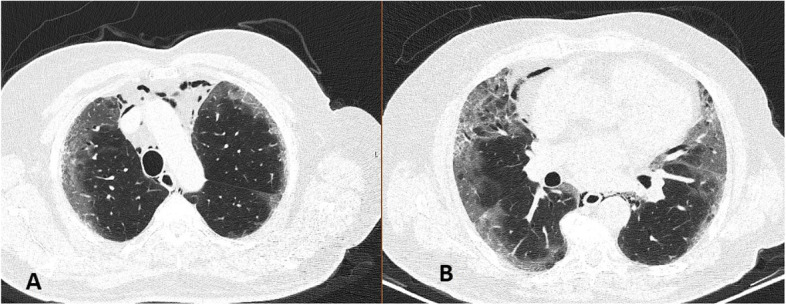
Chest CT obtained at presentation to the ED in case 1, demonstrating mediastinal air (A). It shows bilateral ground-glass opacities and parenchymal consolidation (A–B)
